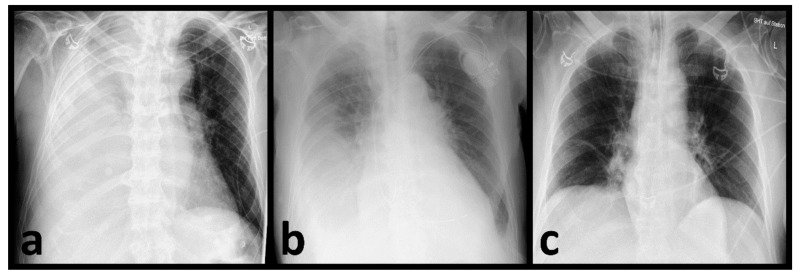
False positive assignments by the AI. Examples of chest radiographs with false positive assignments by the artificial intelligence possibly caused by large pleural effusions (a,b) or a small dystelectasis in the right lower field (c).
